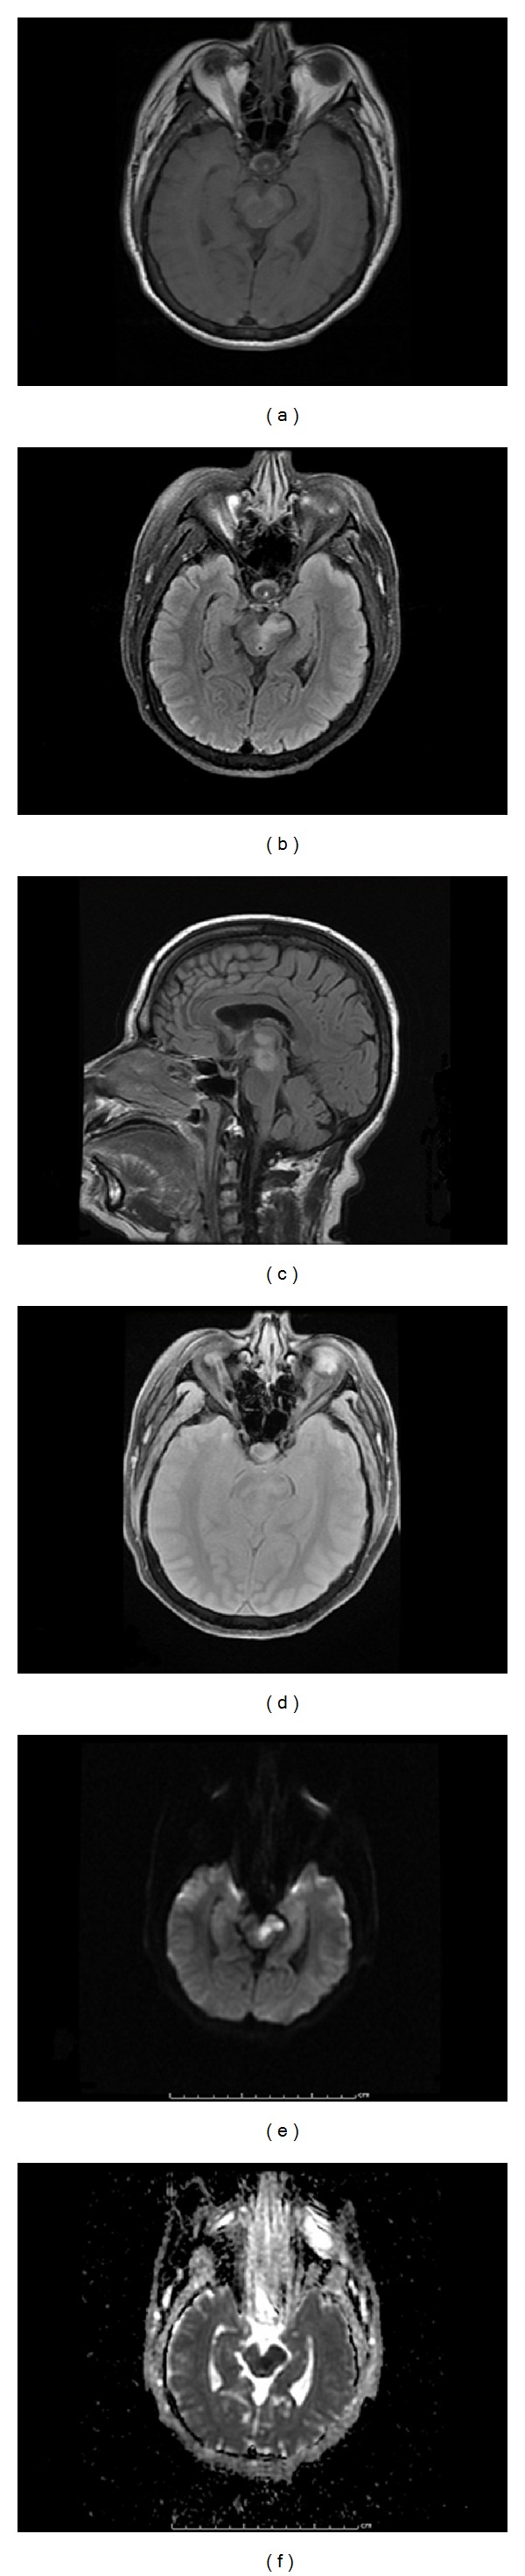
Brain magnetic resonance imaging showing acute infarcts in the thalami, left cerebral peduncle, and mid-brain. (a) Axial T1 weighted image, (b) axial T2 weighted image, (c) sagittal T1 weighted image, (d) axial gradient echo (GRE) image, (e) axial diffusion weighted image, (f) adjusted diffusion coefficient (ADC) image.
